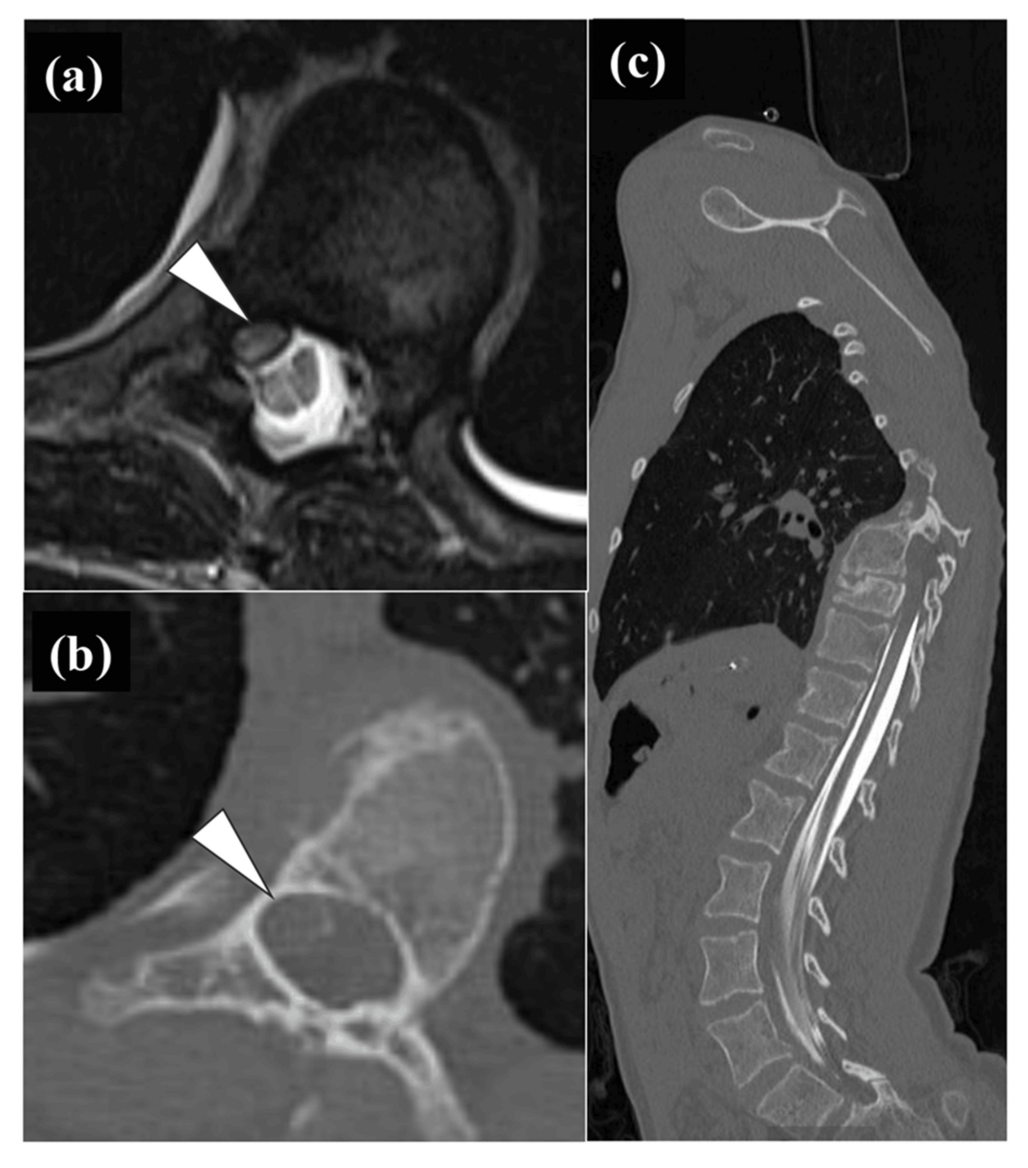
Preoperative magnetic resonance (MR) and computed tomographic myelograhic (CTM) image Axial MR image also showed the deviated and compressed spinal cord (a). CTM image showed that the spinal cord was deviated to the concave side and was compressed from the right pedicle and posterior wall of the vertebral body at T10 level in axial plane (b), and the contrast medium injected into the dural sac from L4/5 level did not flow cephalad from T10 in sagittal plane (c).The white triangles in (a) and (b) indicated the dural sac and spinal cord.
